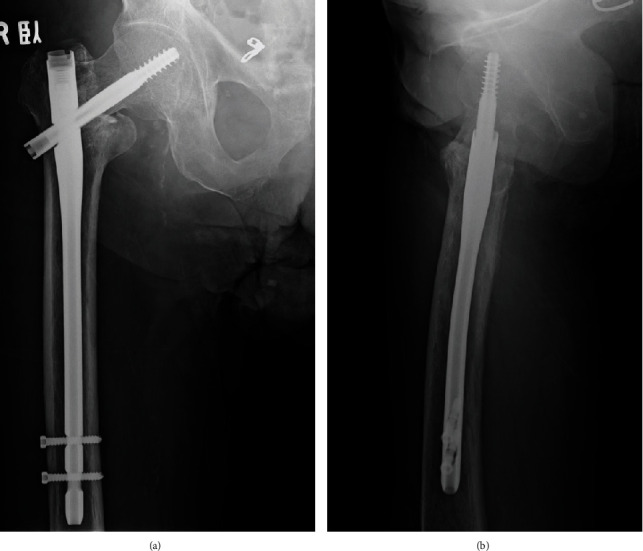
Radiographs after the osteosynthesis of the right trochanteric fracture. Anteroposterior (a) and lateral (b) views of the right femur showing insufficient reduction and poor placement of the lag screw after implantation of a gamma 3 long nail.
